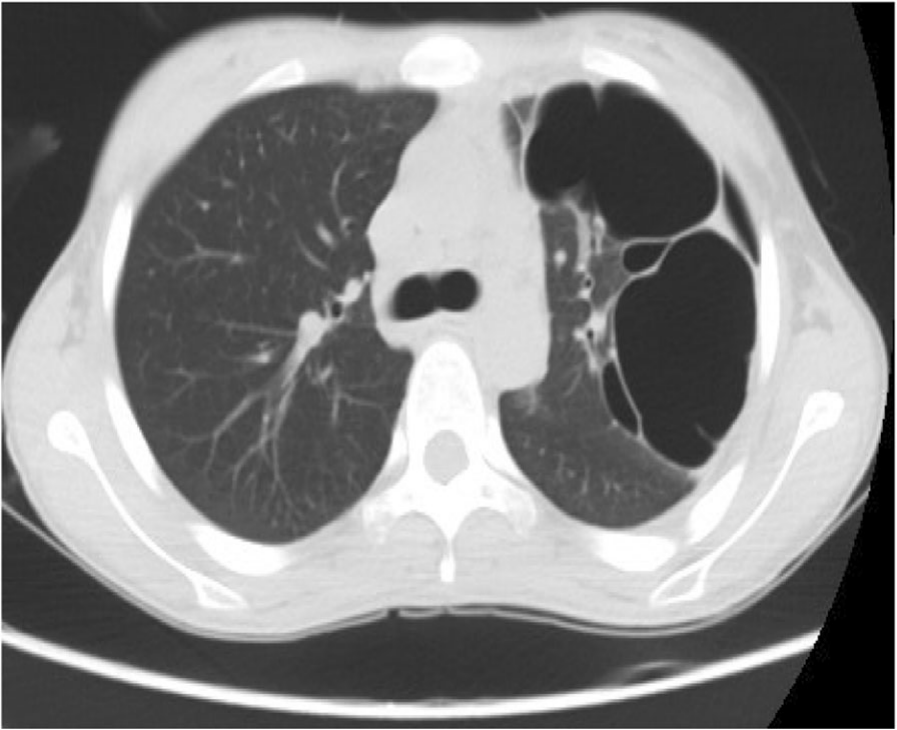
Computed tomography image 1 month after surgery: consolidation improved and cystic lesions did not decrease
